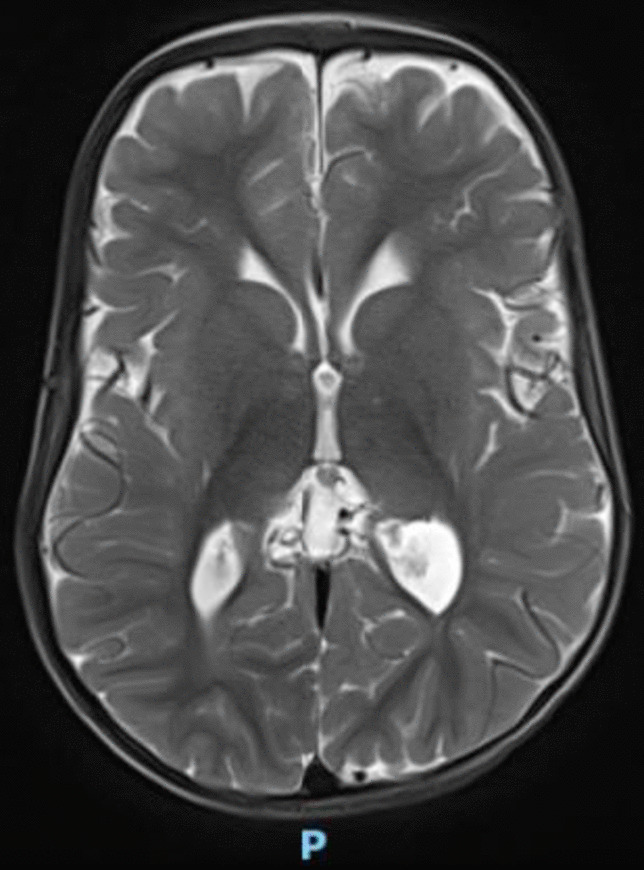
Normal magnetic resonance imaging of the brain
